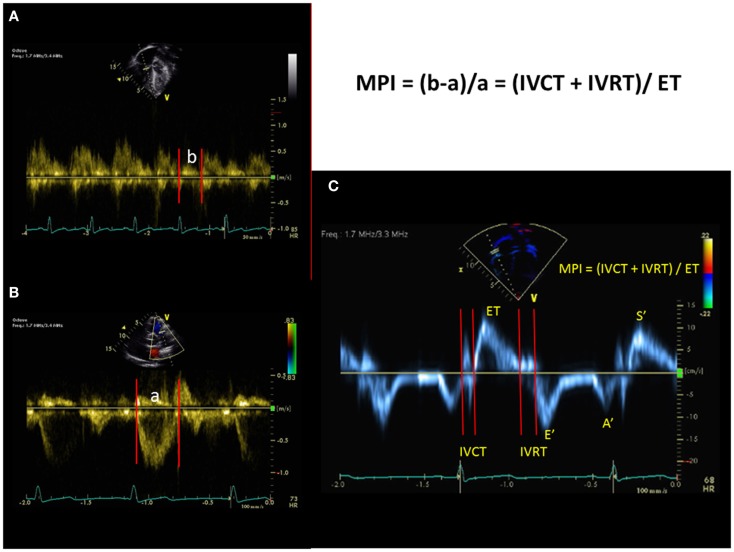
The myocardial performance index (MPI) can be obtained from the spectral Doppler of tricuspid inflow and pulmonary outflow velocities [top left and bottom left, (A,B)] or can be obtained from tissue Doppler imaging (C) at the lateral annulus of the tricuspid valve. IVCT = isovolumic contraction time, IVRT = isovolumic relation time, and ET = ejection time. Tissue Doppler imaging of the tricuspid valve. *S′*= systolic velocity, *E′* = early diastolic velocity, *A′* = late diastolic velocity.
